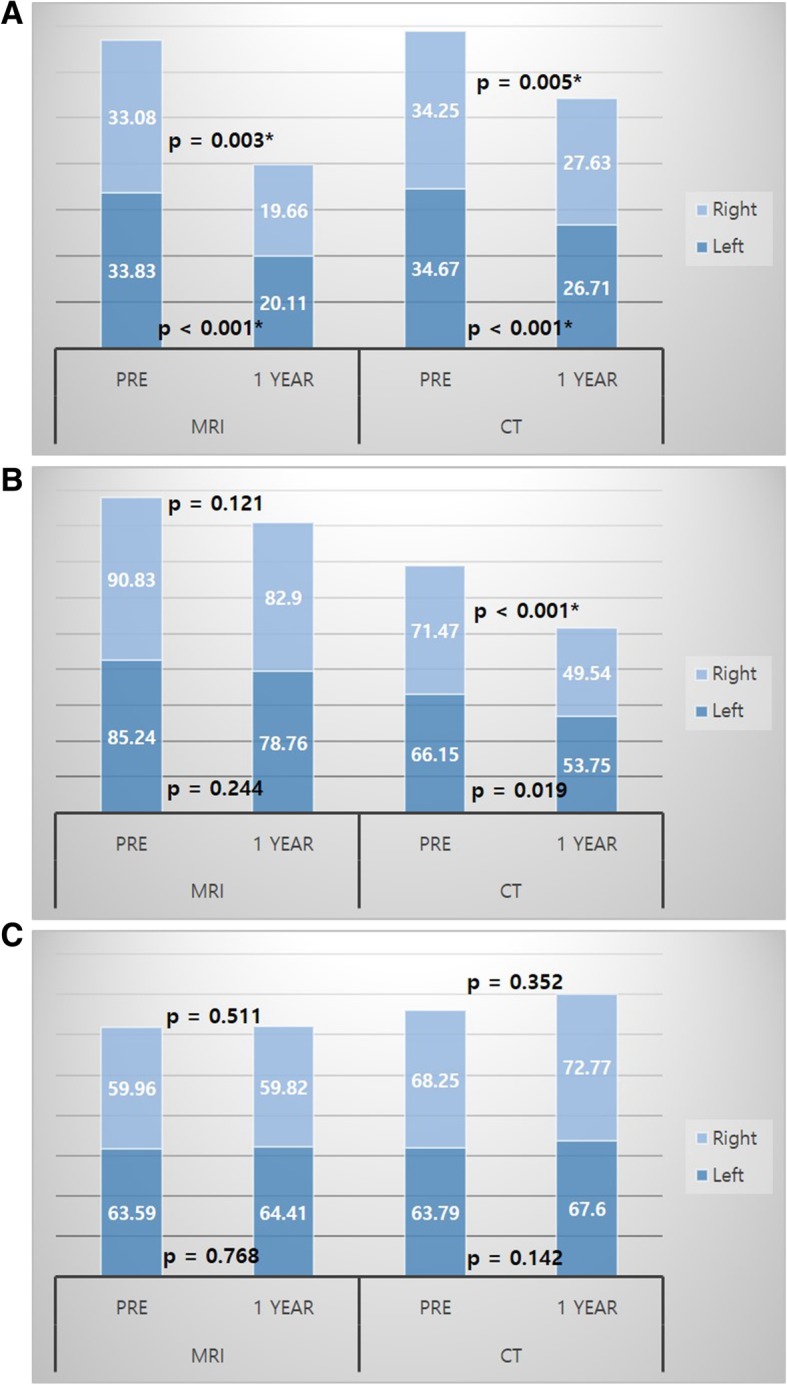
Histograms of the sum of right and left paraspinal muscles on MRI and CT. a Volume of the multifidus muscles. b Volume of the erector spinae muscles. c Volume of the psoas muscles
